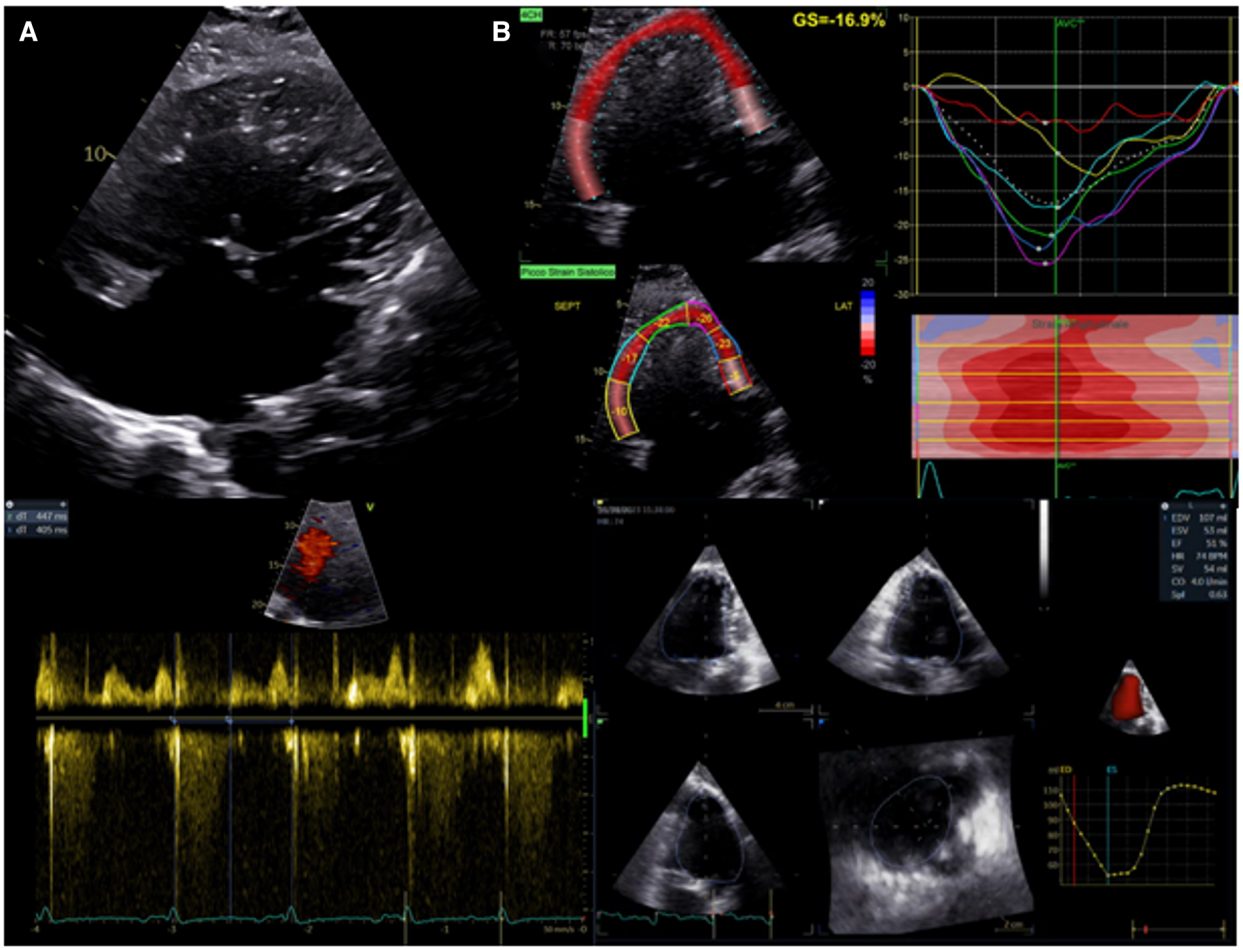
Evaluation of systolic function in patients with univentricular heart after fontan procedure. (A) Upper panel: apical 2D view. Lower left panel: atrioventricular systolic to diastolic duration ratio. (B) Upper panel: univentricular speckle tracking analysis. Lower right panel: 3D ejection fraction evaluation.
